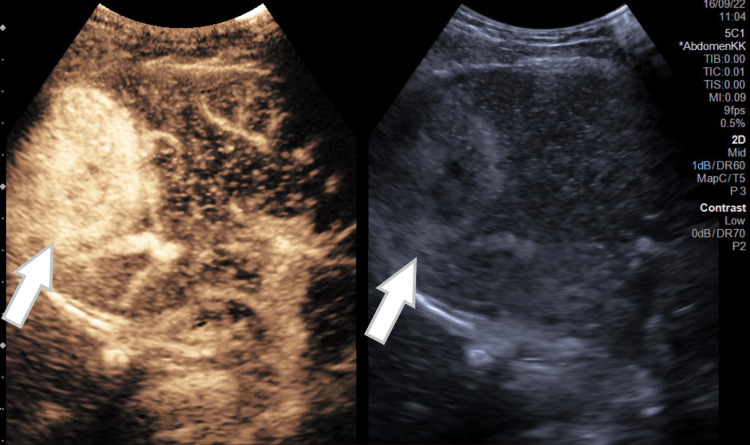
Contrast-enhanced ultrasound (CEUS) of the liver; white arrow shows a segment VII lesion
